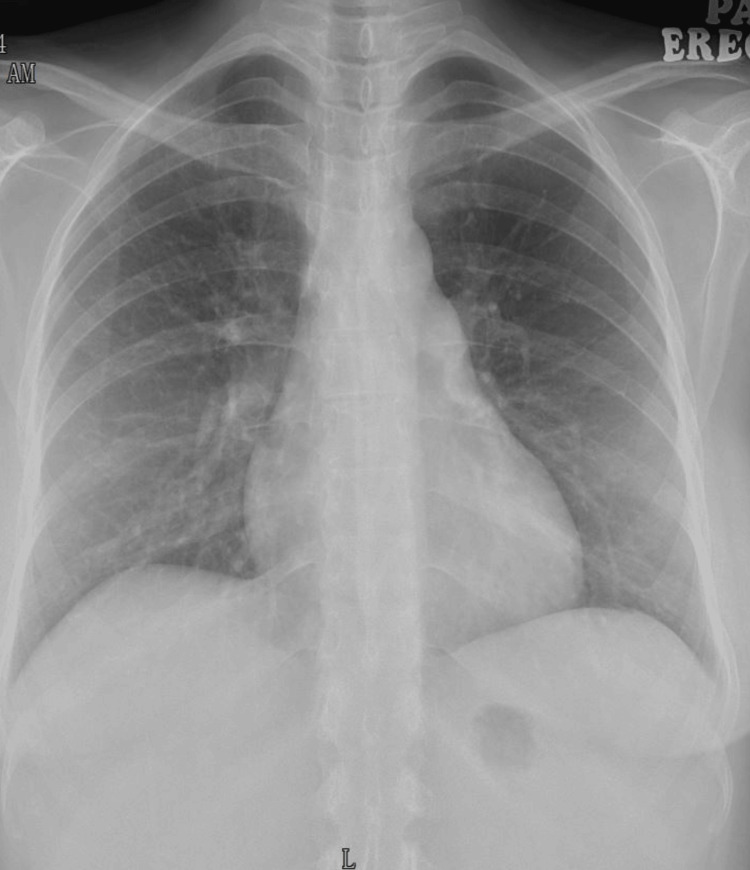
Chest X-ray on admission Chest radiograph on admission showing clear lung fields.
